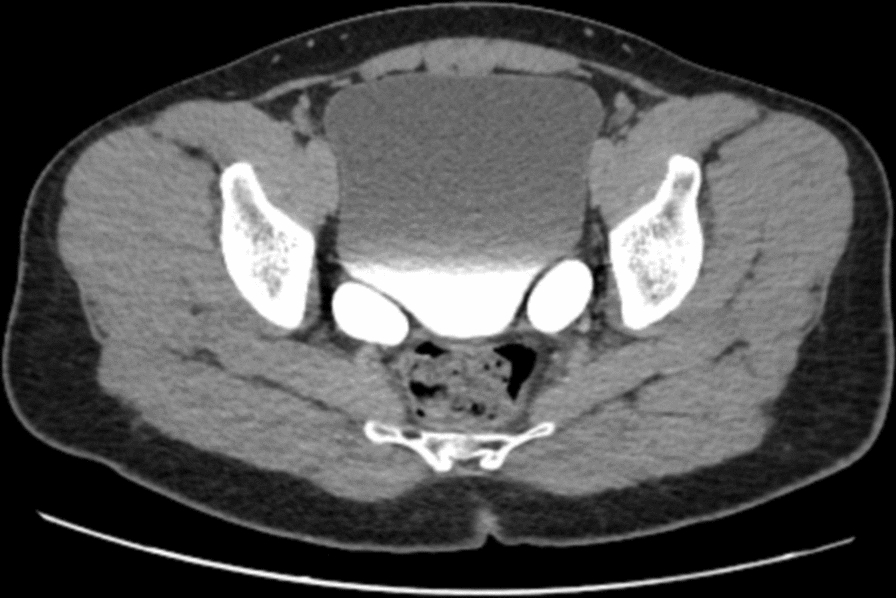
A contrast-enhanced computed tomography of kidneys, ureters, and bladder (axial section). Urinary bladder is optimally distended, thin-walled, underfilled with contrast without filling defect. Prostate gland is normal in size. Seminal vesicles are normal in appearance. No bowel obstruction or collection. No lymphadenopathy and no free fluid in abdomen and pelvis. No large mass seen in the liver, kidneys, spleen, the adrenal glands and pancreas. No destructive bony lesions seen in the visualized bony structures
